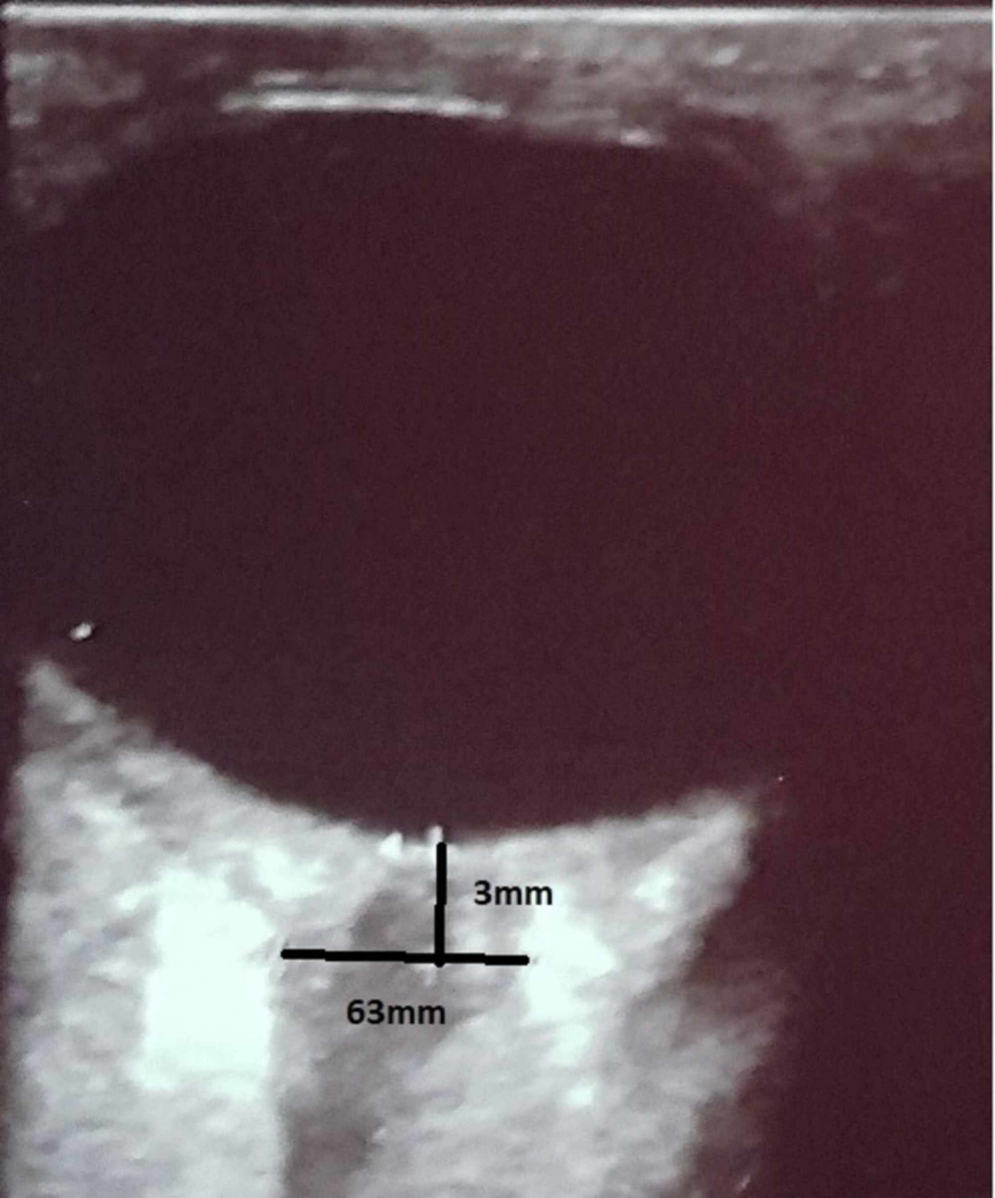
The patient’s enlarged ONSD measured 3 mm posterior to the retina with a high-frequency ultrasound probe ONSD: optic nerve sheath diameter
